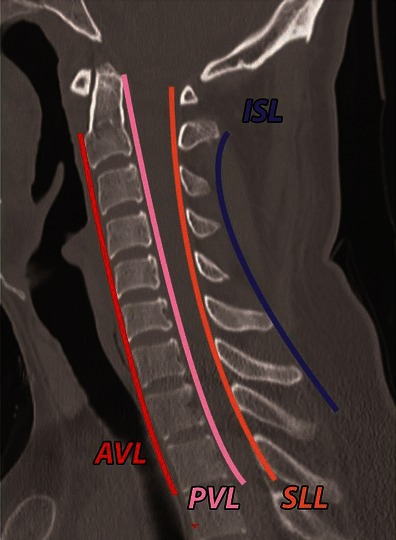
Normal alignment of the spine as seen on mid-sagittal CT: anterior vertebral line (*AVL*, *red*)—connecting the anterior cortices of the vertebrae; posterior vertebral line (*PVL*, *pink*)—connecting the posterior cortices of the vertebrae; spinolaminar line (*SLL*, *orange*)—connecting the base of the spinous processes at the spinolaminar junction; interspinous line (*ISS*, *blue*)—connecting the tips of the spinous processes. All of these lines should be gently curved, smooth and continuous
